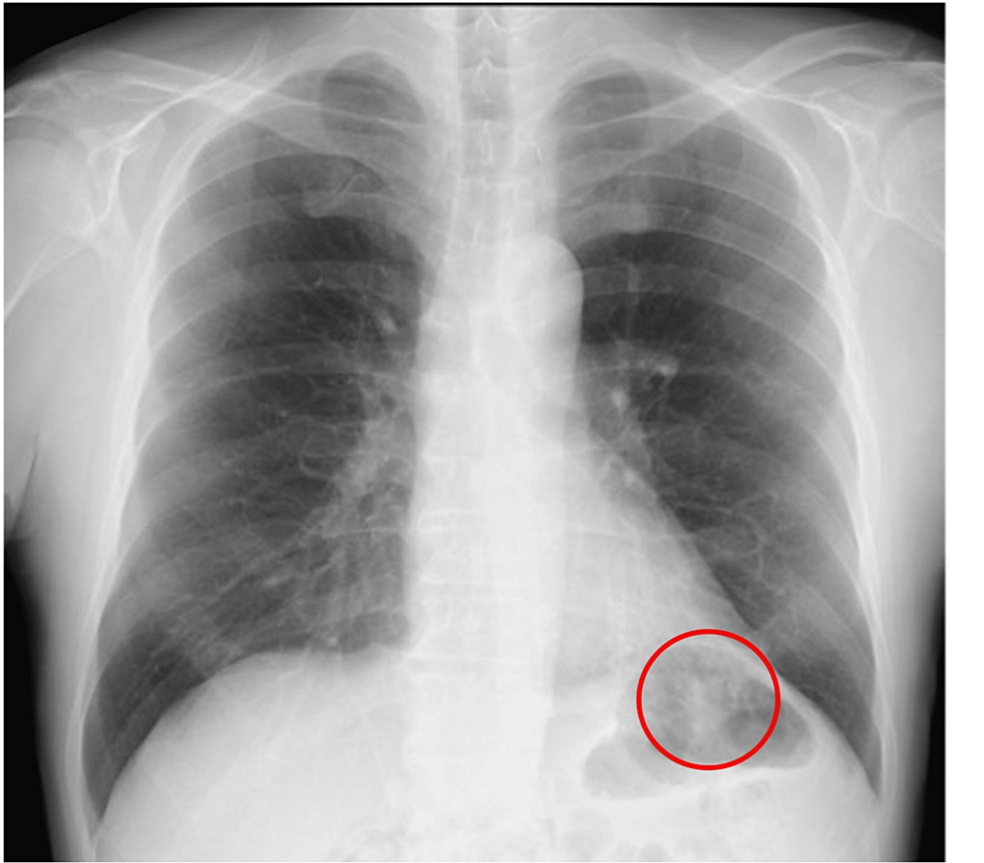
Chest radiograph on admission for a bronchoscopy An infiltrative shadow in the left lower lung field was demonstrated (red circle).
